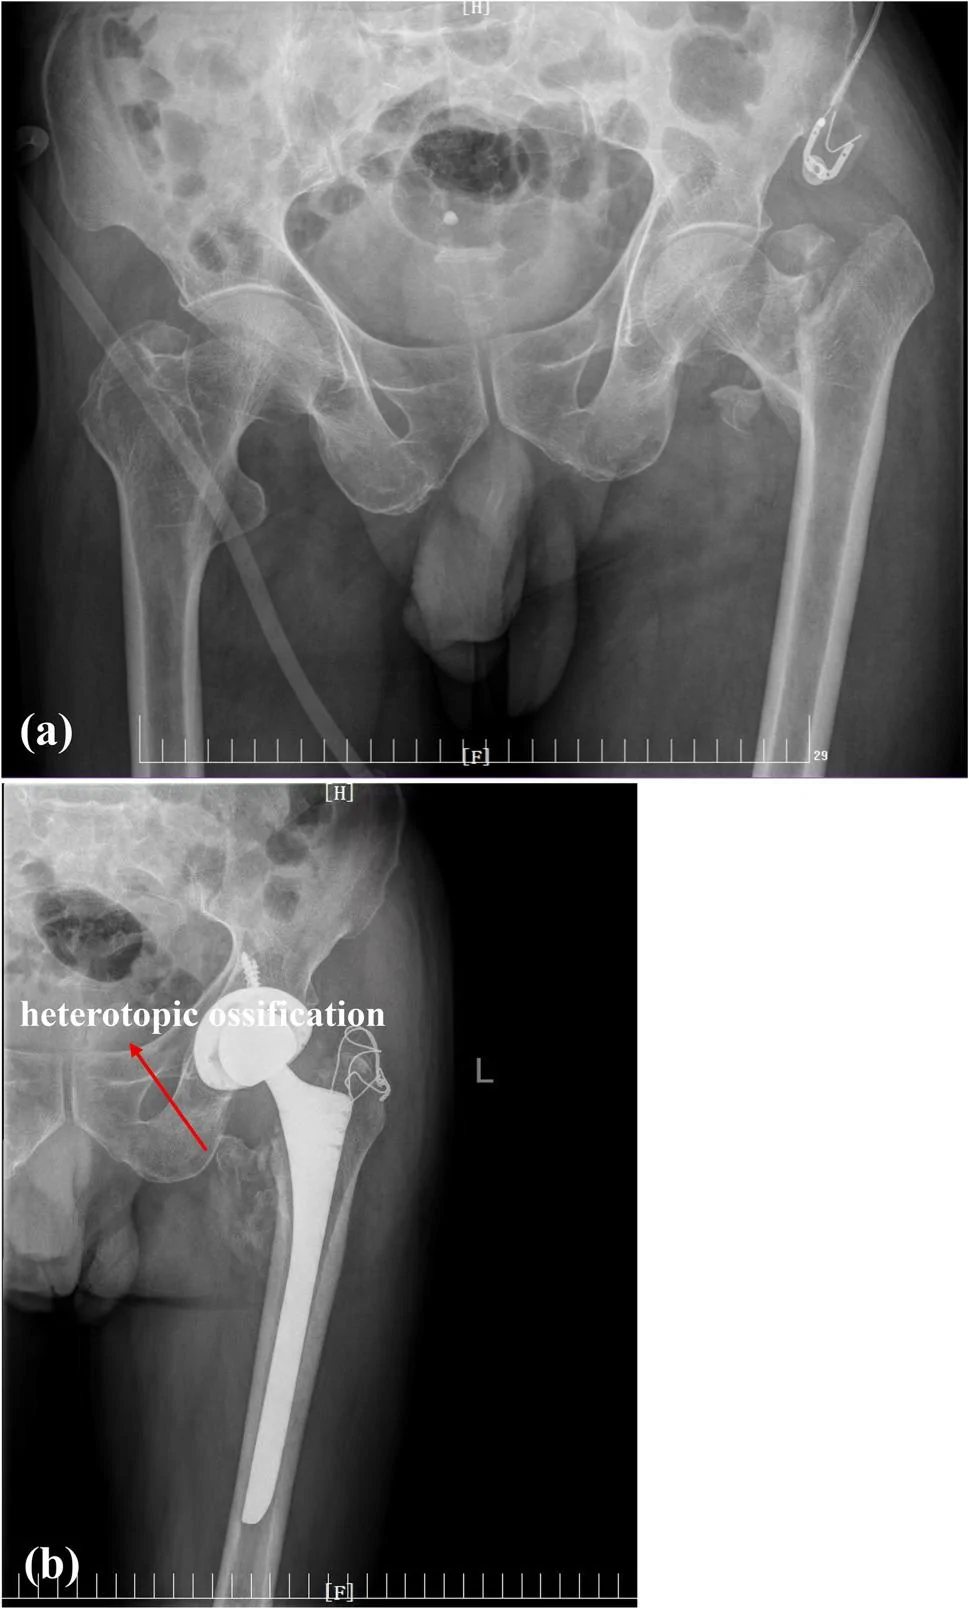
Images of a male patient aged 70–80 years with left hip femoral intertrochanteric fracture. a Postoperative radiograph. b Radiograph 3 years after surgery: severe periprosthetic heterotopic ossification
